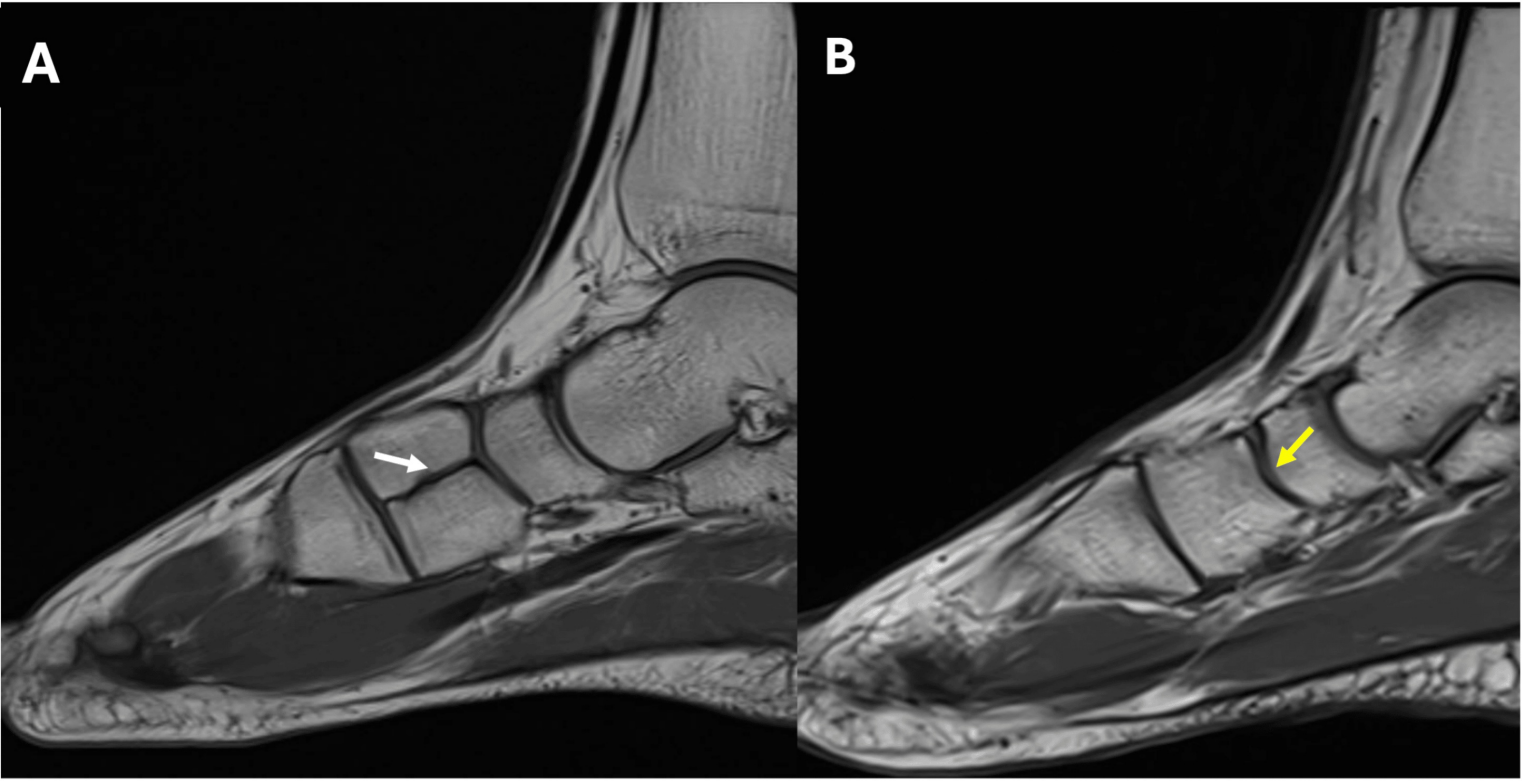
Sagittal T1 MRI: (A) showing dorsal and plantar components of bipartite medial cuneiform (white arrow) articulating with the navicular bone and the base of the first metatarsal bone compared to (B) showing the normal tarsal bone anatomy in a patient without anatomical variation (yellow arrow).
